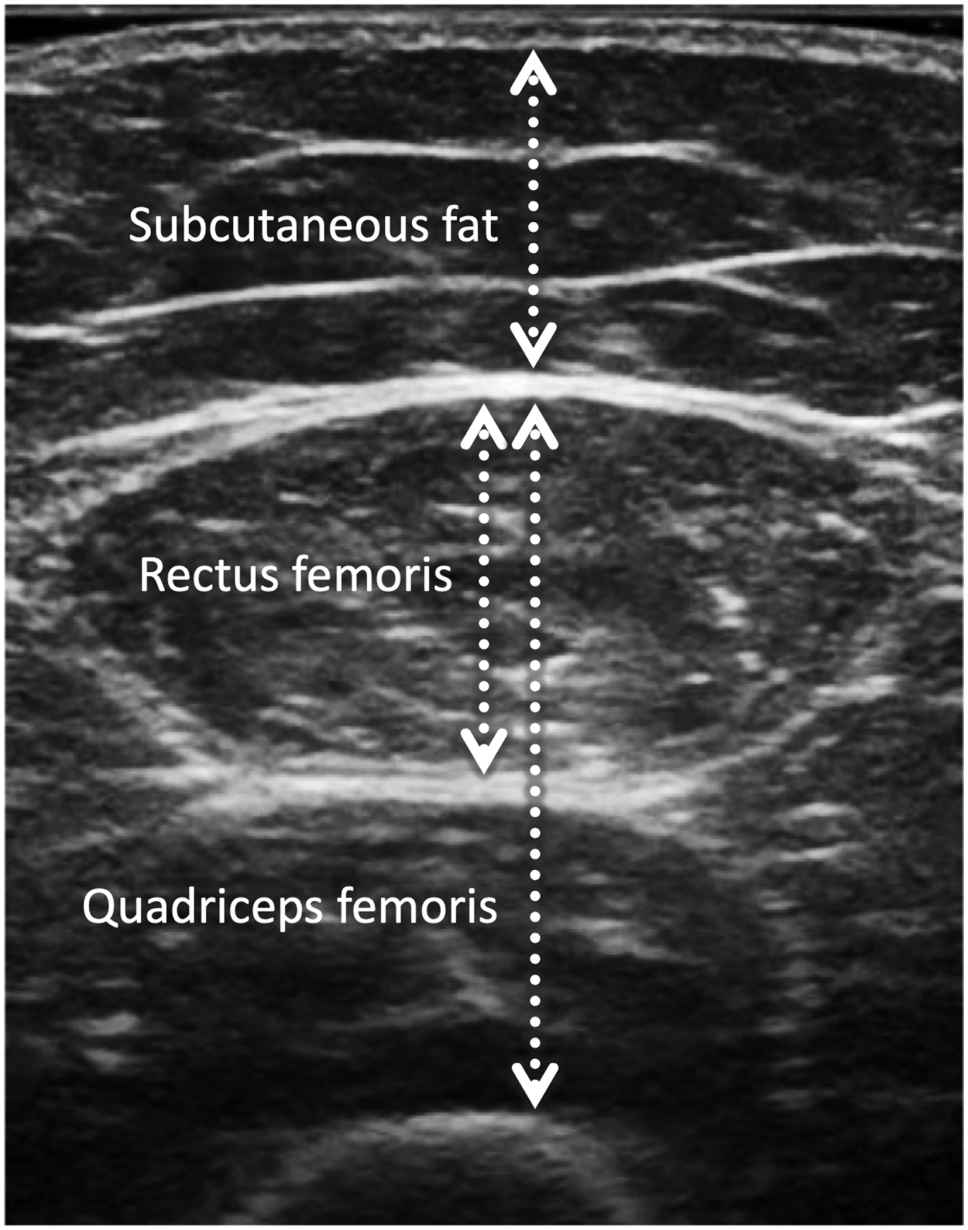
Ultrasonography measurement of subcutaneous fat thickness, rectus femoris thickness, and quadriceps muscle thickness
